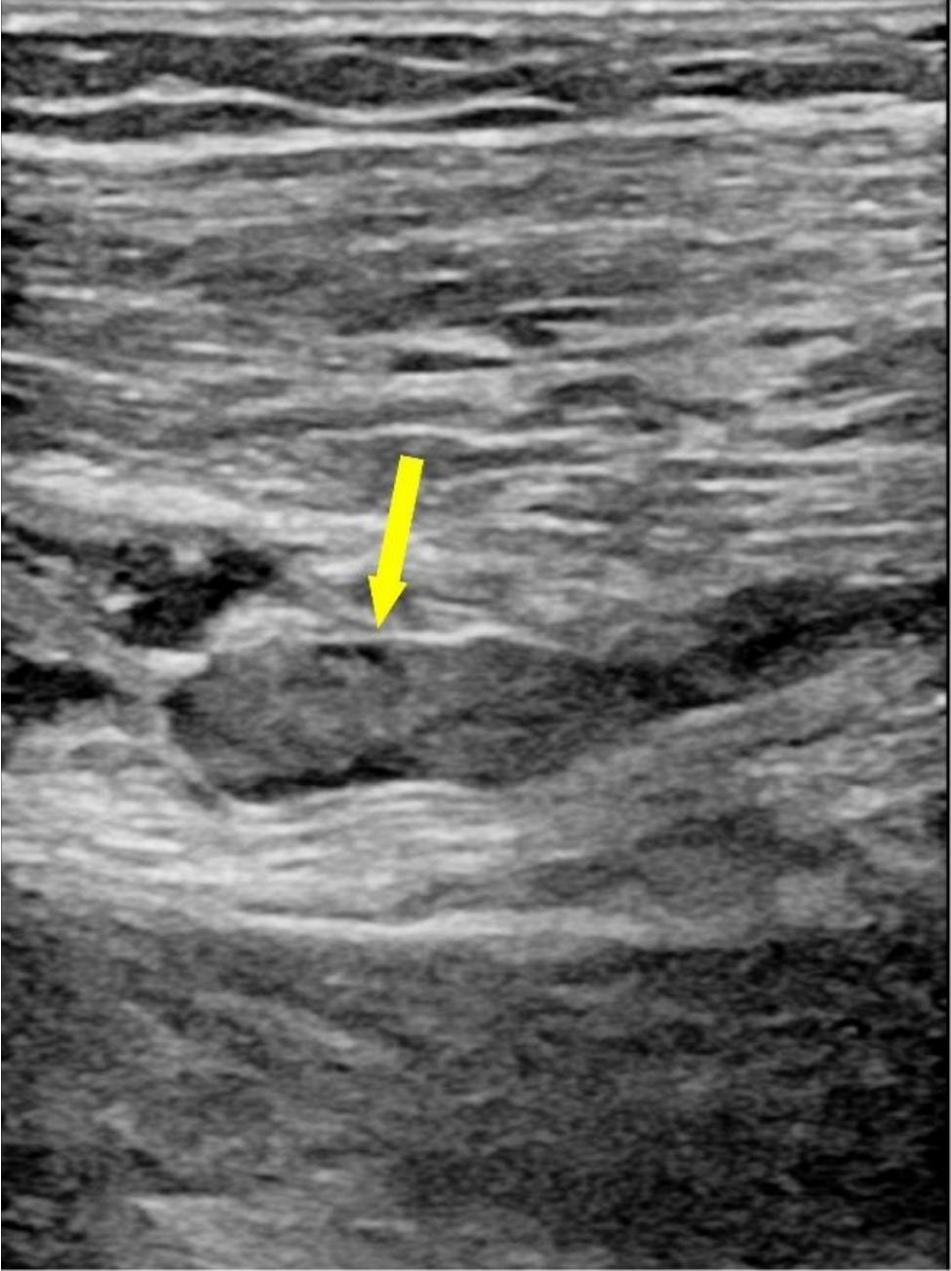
DVT in Doppler ultrasonography (Yellow arrow)
